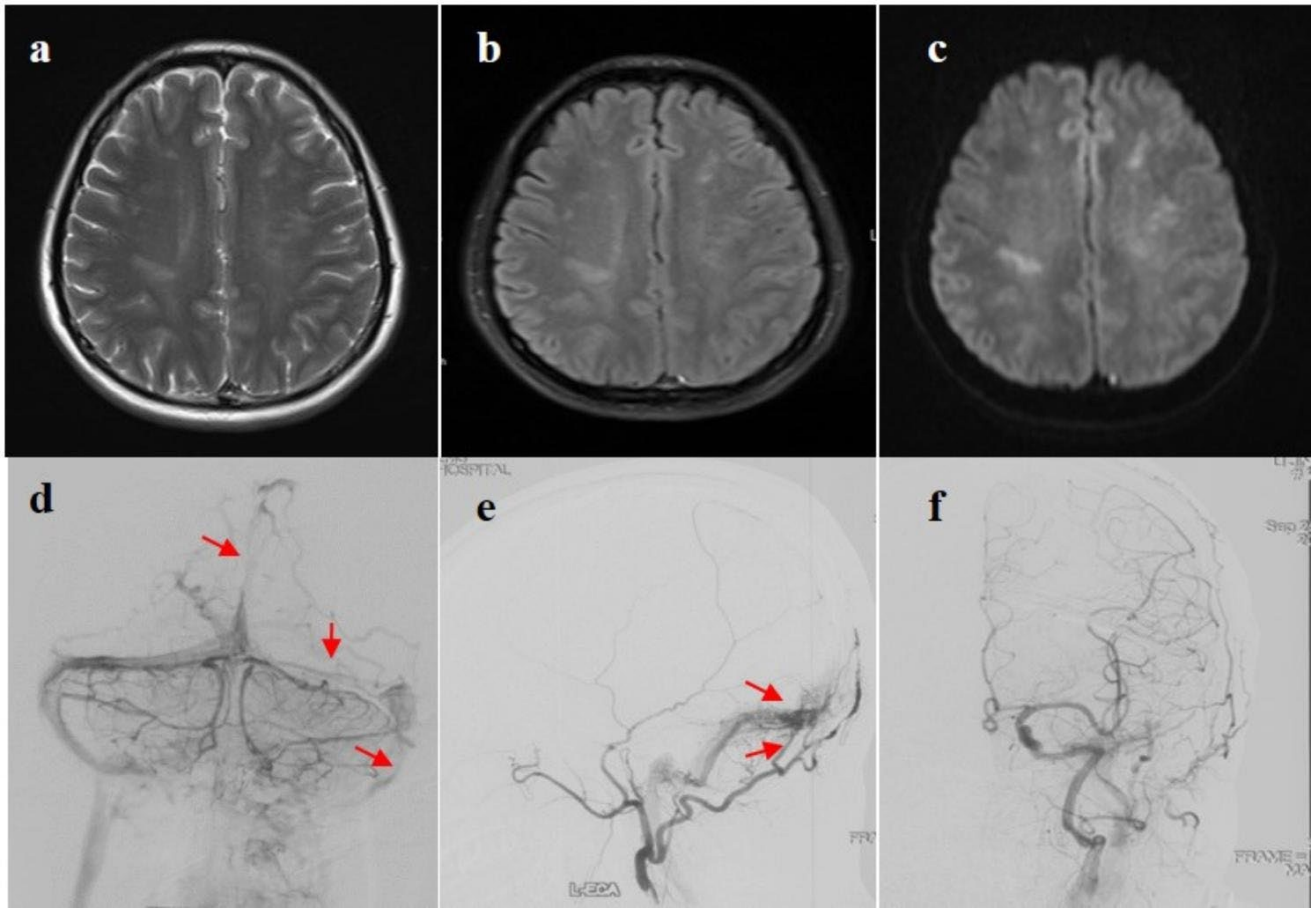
Brain MRI showed abnormal signal in bilateral cerebral hemisphere. a, b, c: T2-, FLAIR, DWI-weighted images, showing hyperintensity, respectively. The venous phase of DSA (d) showed poor visualization of superior sagittal sinus, left transverse sinus and left sigmoid sinus were not indistinct (red arrows); The follow-up of DSA 3 months later (e) revealed a DAVF in the region of left lateral sinus supplied by the left occipital artery (red arrows); DAVF disappeared completely after embolization (f)
